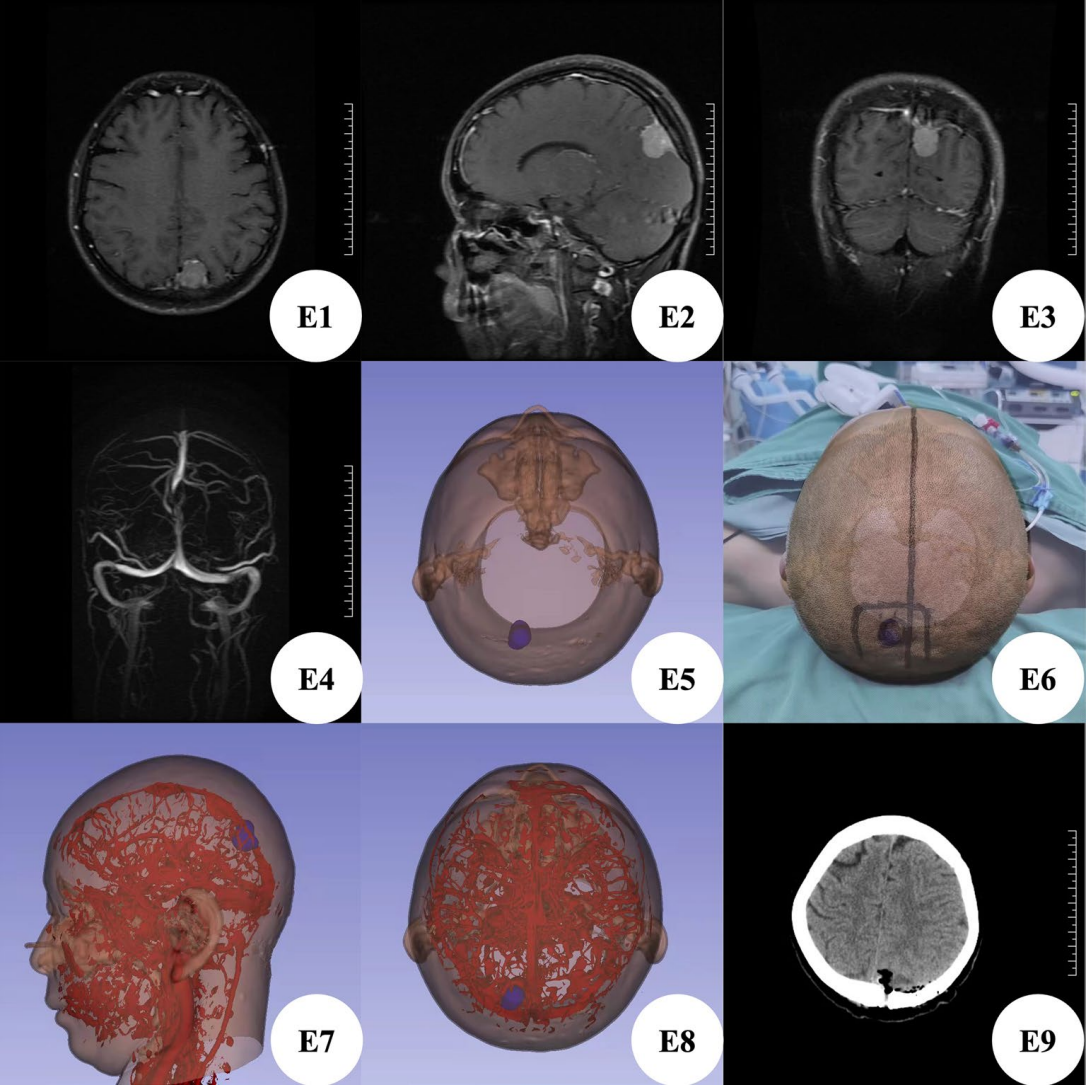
(E1–E3) Brain MRI enhancement showed left parietal meningioma; (E4) brain MRV showed that the posterior part of intracranial superior sagittal sinus became narrower; (E5) 3D Slicer was used to reconstruct the left parietal meningioma image; (E6) the Apple mobile phone APP MosoCam was used to design the left parietal space occupying projection and surgical incision; (E7,E8) 3D Slicer was used to reconstruct the image of the relationship between intracranial vessels and left parietal meningioma; (E9) postoperative brain CT showed that the tumor was resected cleanly without delayed bleeding.
